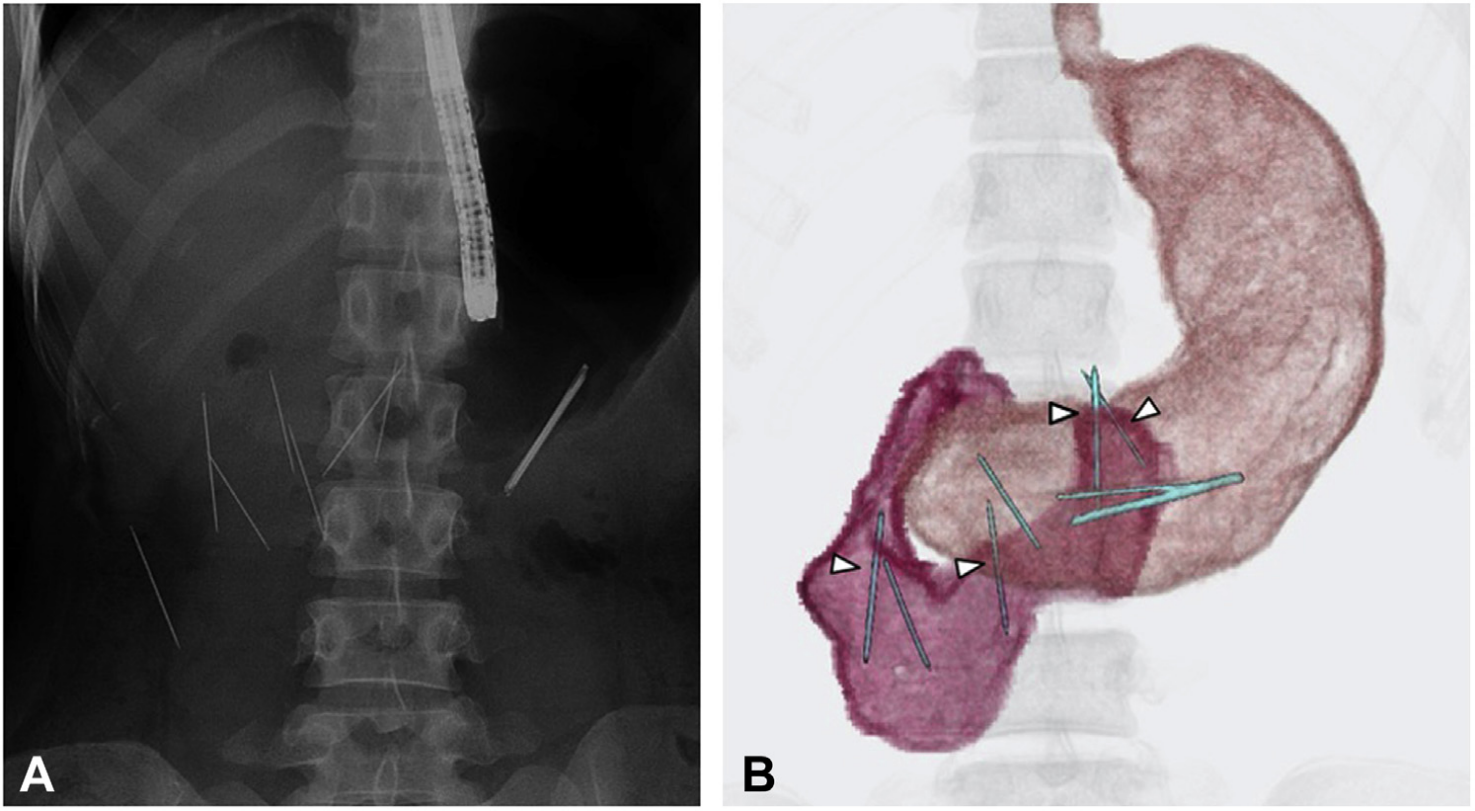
A, Fluoroscopic images showing multiple sewing needles in the stomach and duodenum. B, Upper GI images by CT reconstruction show multiple needles in the stomach and duodenum. Some are penetrating the duodenal wall (*arrowheads*).
